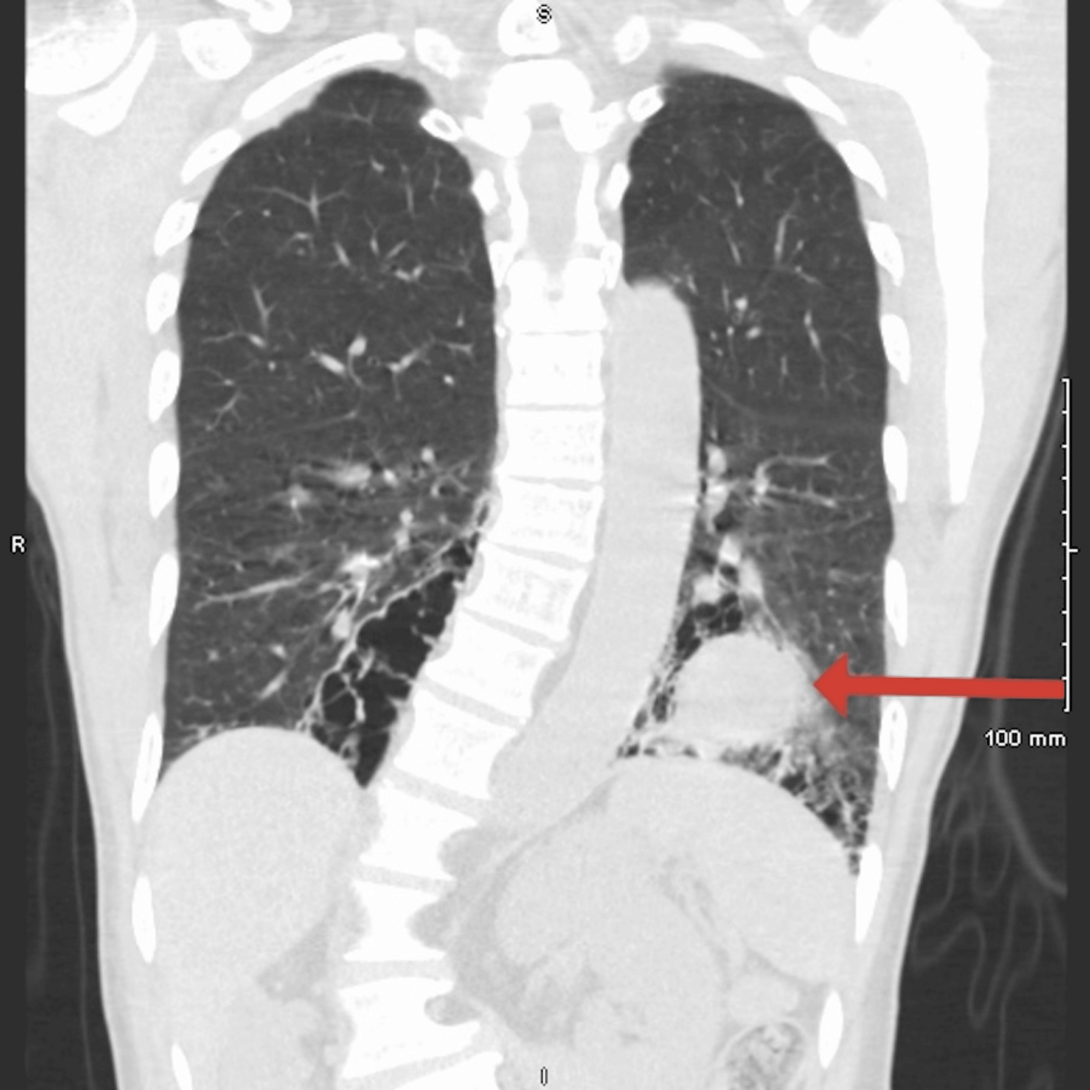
Non-contrast CT image of the chest demonstrating a solid nodule in the left lower lobe, as indicated by the red arrow
